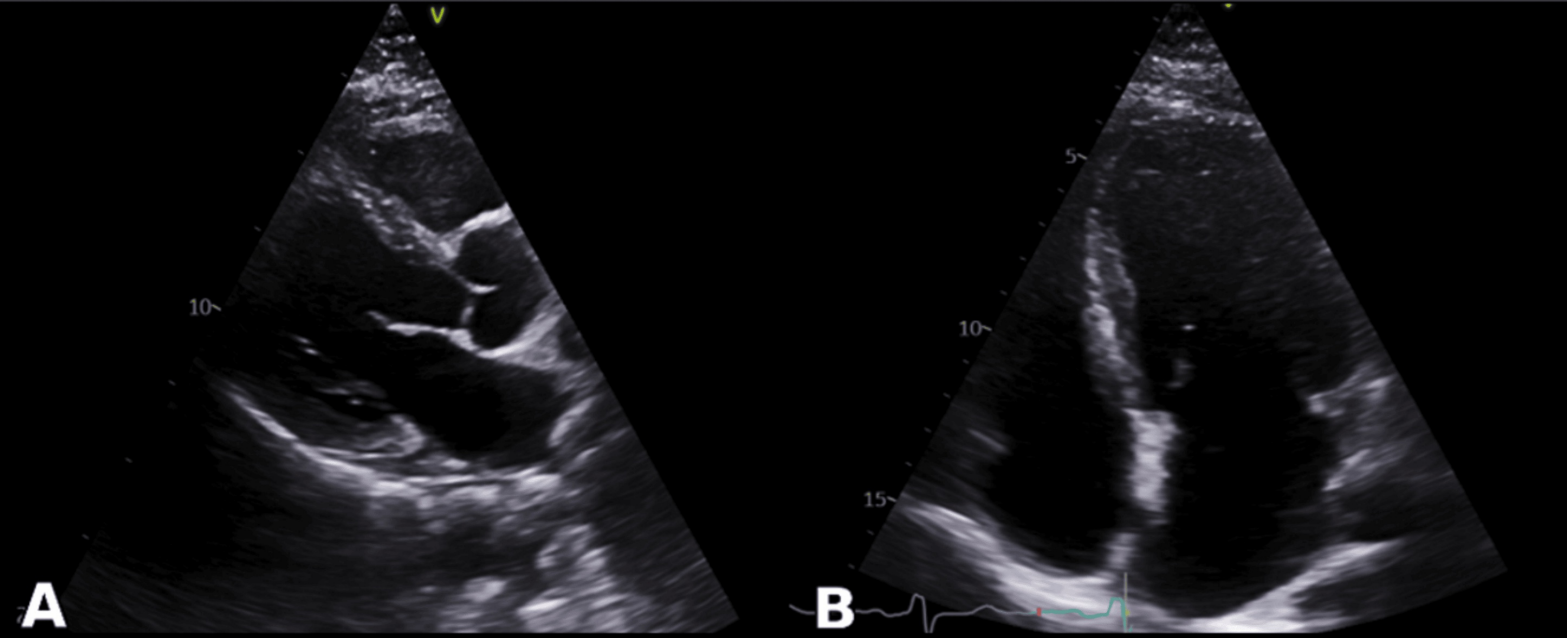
(A) Echocardiographic parasternal long-axis (PLAX) view and (B) Apical four-chamber (A4C) view showing normal left ventricular cavity and normal mitral and aortic valves structure with normal left ventricular wall and septal thickness.
